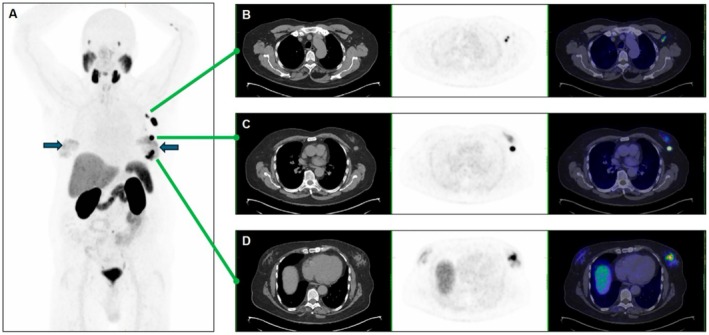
A: Whole body 68Ga‐PSMA PET/CT scan demonstrating multiple PSMA‐avid lesions in the left breast on background of mildly PSMA‐avid gynecomastia (solid arrows). Physiologic PSMA activity in the lacrimal glands, salivary glands, liver, spleen, kidneys, bowel, ureters, and urinary bladder. Panel B: Small PSMA‐avid left axillary nodes on CT, PET and fused images. Panels C & D: Multifocal PSMA‐avid left breast lesions on CT, PET and fused images. The combination of a focal breast lesion with ipsilateral axillary nodal uptake created an imaging pattern closely resembling a primary breast carcinoma with regional nodal drainage.
